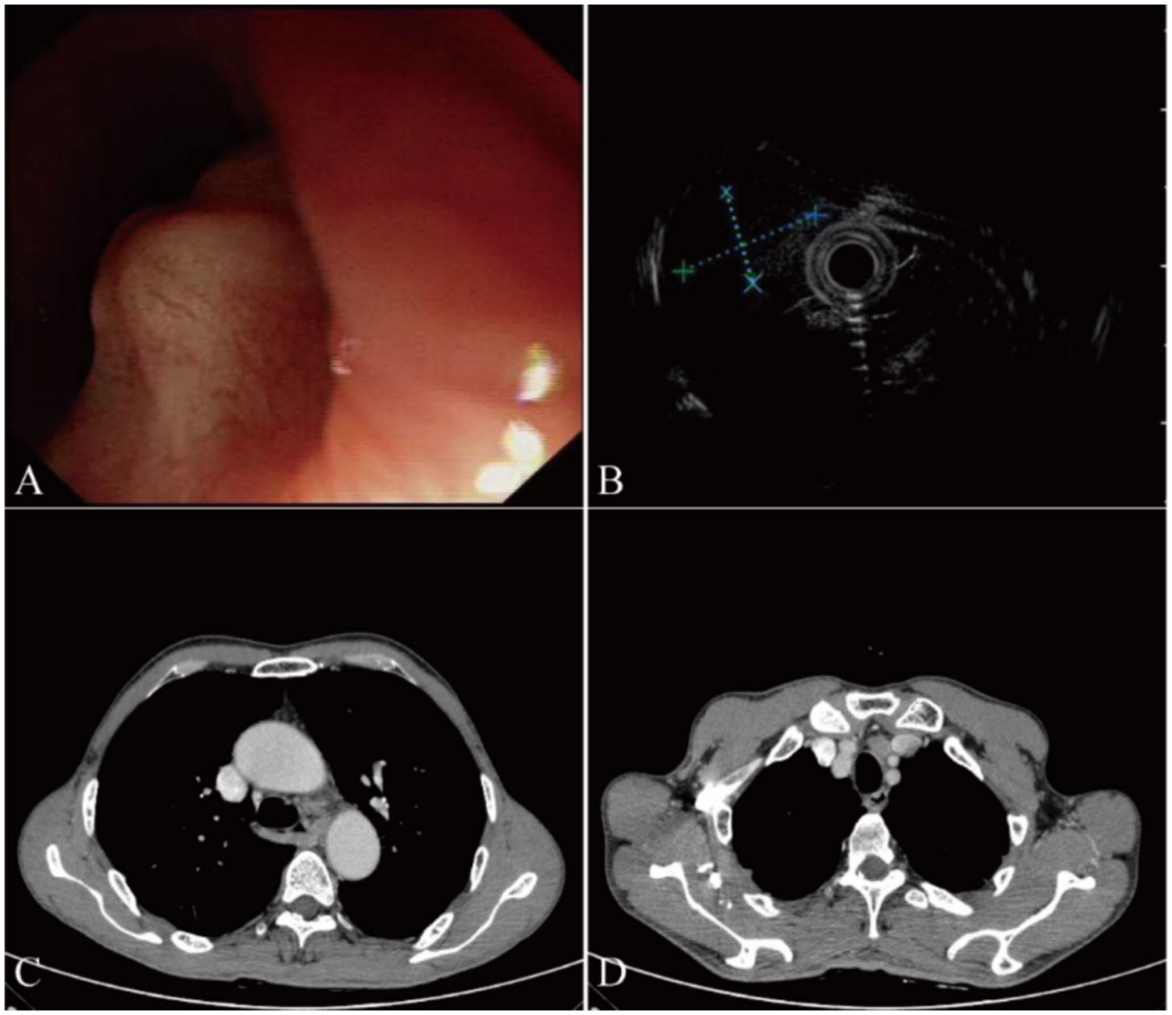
Endoscopic examination and computed tomography (CT) results in our case. (A) White light endoscopy shows a smooth subepithelial elevation measuring approximately 1.5 cm in diameter, located 27 cm from the incisors along the left esophageal wall. The lesion appears well-defined and does not cause significant luminal narrowing. (B) Endoscopic ultrasonography (20 MHz) reveals an ovoid hypoechoic mass originating from the submucosal layer. The tumor measures approximately 9.1 mm × 6.1 mm in size, with well-defined borders and no signs of infiltration into surrounding structures. (C) Enhanced chest CT scan demonstrates a slight thickening of the middle segment wall of the esophagus, suggesting the presence of a submucosal lesion. (D) Enlarged lymph nodes anterior to the main bronchus are visible on the CT scan, indicating possible regional lymphadenopathy.
